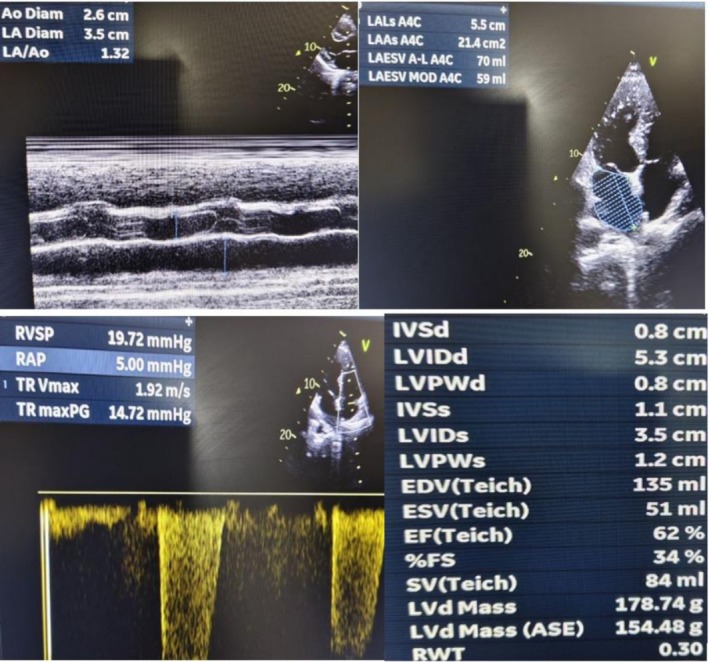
Echocardiogram of 52 years old male with meningitis complicated with ischemic stroke and failed thrombolysis showing—Normal Echocardiogram.
